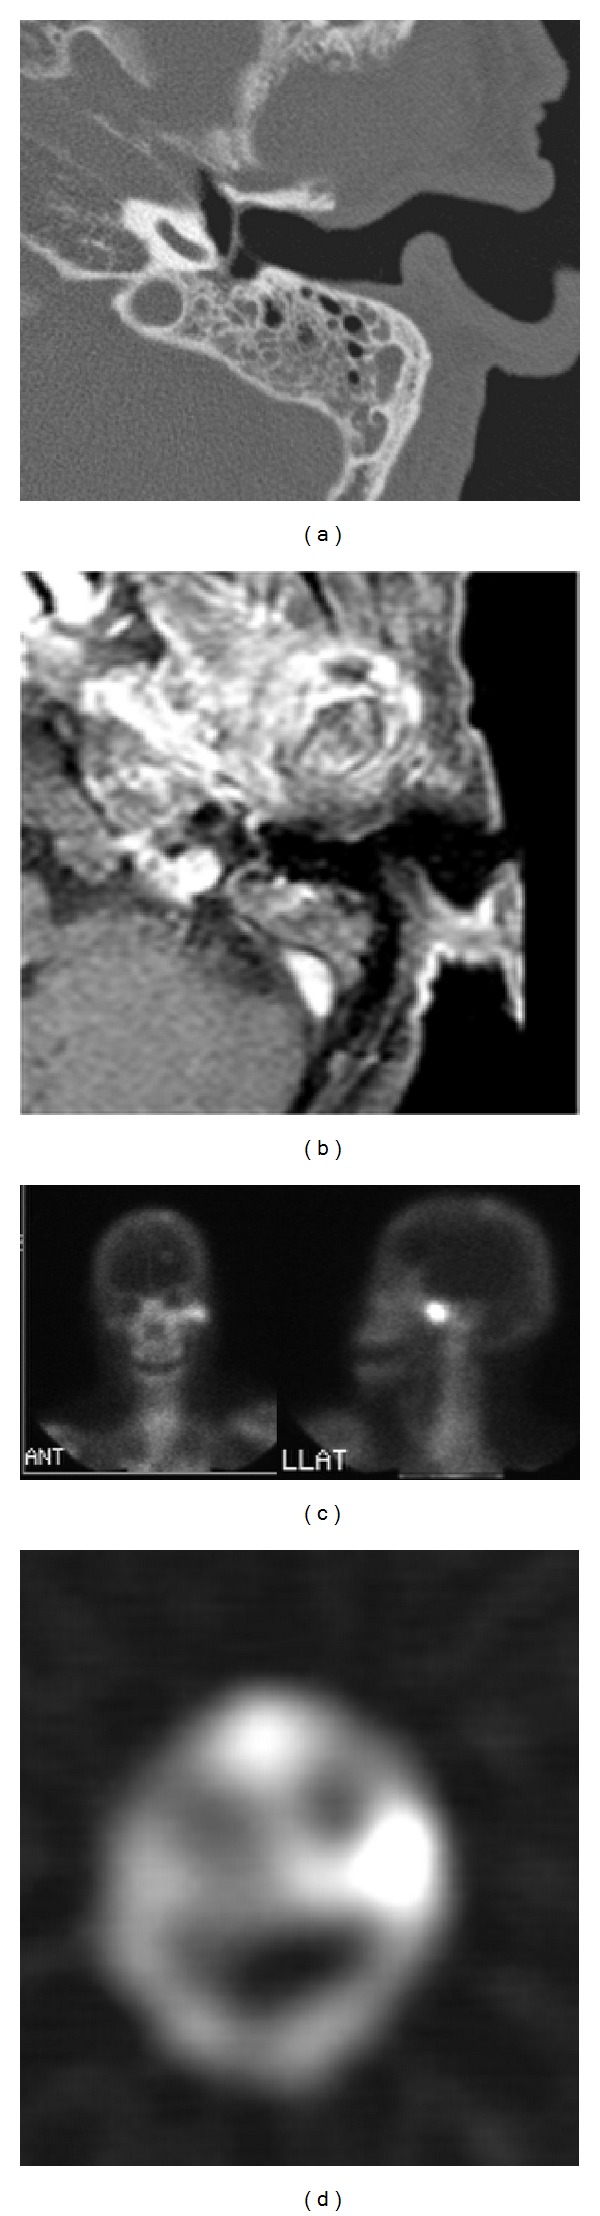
Images of a patient of temporal bone osteomyelitis without history of DM. (a) CT. (b) Contrast-enhanced T1-weighted MR. (c) Tc99m bone scan. (d) Gallium scan. See text for details.
